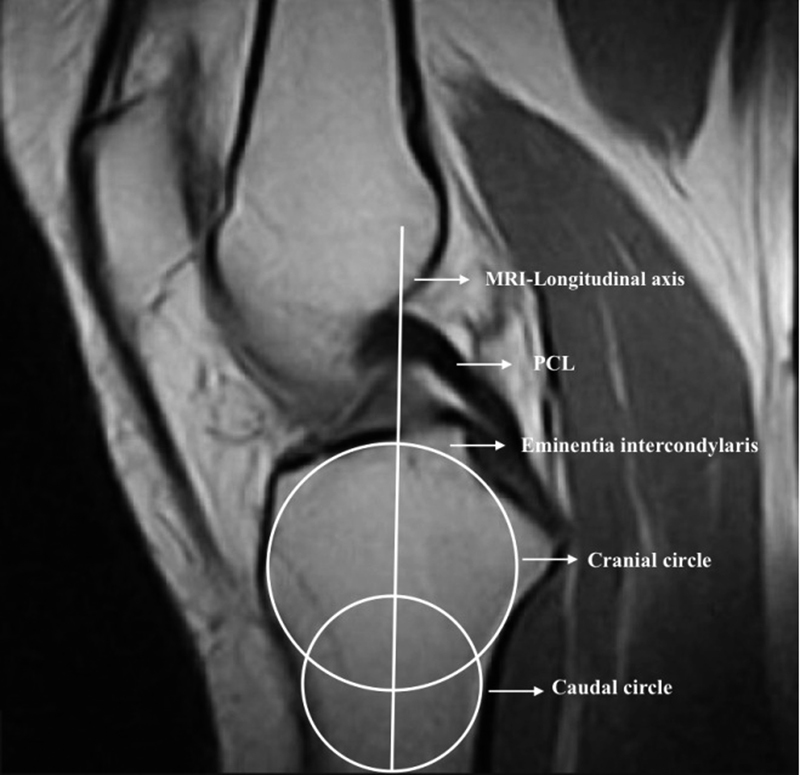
The measuring technique described by Hudek et al. In the first step, the central sagittal image is identified in which the tibial attachment of the posterior cruciate ligament (PCL) and the intercondylar eminence is seen. Subsequently, two circles are placed in the tibial head. A cranial circle which touches the anterior, posterior, and cranial cortex and a caudal circle which touches the anterior and posterior cortex. The center of the caudal circle is hereby positioned on the circumference of the cranial circle. The line connecting the centers of both circles is defined as the magnetic resonance imaging (MRI) longitudinal axis of the tibia and is propagated through the sagittal MRI series. The anatomical center of the lateral tibial plateau is identified on axial slides.
